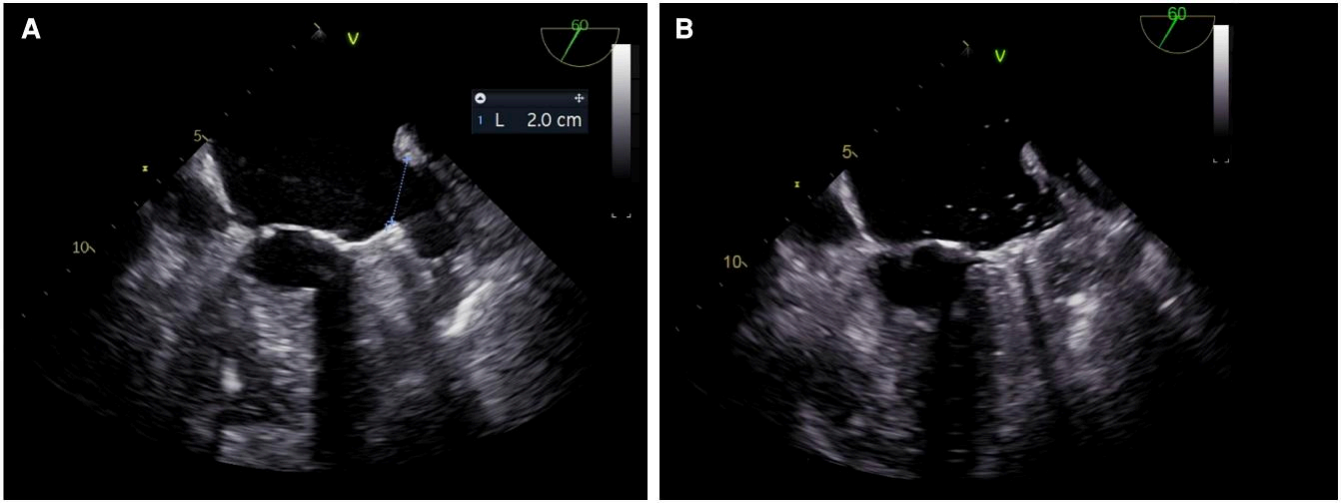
Pre-procedural (*A*) and post-procedural (*B*) 2D transoesophageal echocardiography. Left atrial appendage ostium measured 20 mm.
